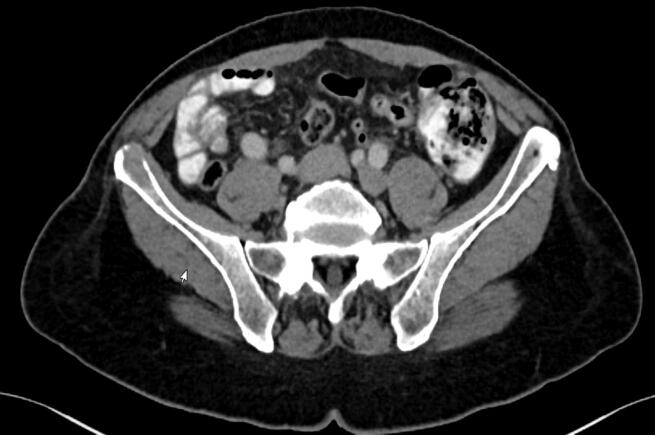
Example case – Diagnostic, axial CT image for “dummy treatment plan” creation. Abbreviations: CT = Computer tomography.Notes: Based on a diagnostic CT image, the treating radiation oncologist indicated the lesion to treat; treatment planners developed a dummy treatment plan.
